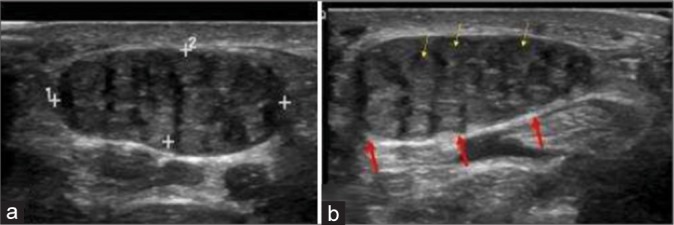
A 10-year-old girl with bilateral fibrolipomatous hamartomas of median nerve who presented with swelling around bilateral wrist and distal forearm. (a, b) Gray scale ultrasound images of proximal volar aspect of bilateral hands showing characteristic coaxial encasement of median nerves by solid echogenic heterogeneous substratum (red arrows). Note thin yellow arrows highlighting a few nerve fascicles on the background of fibrofatty replacement.
